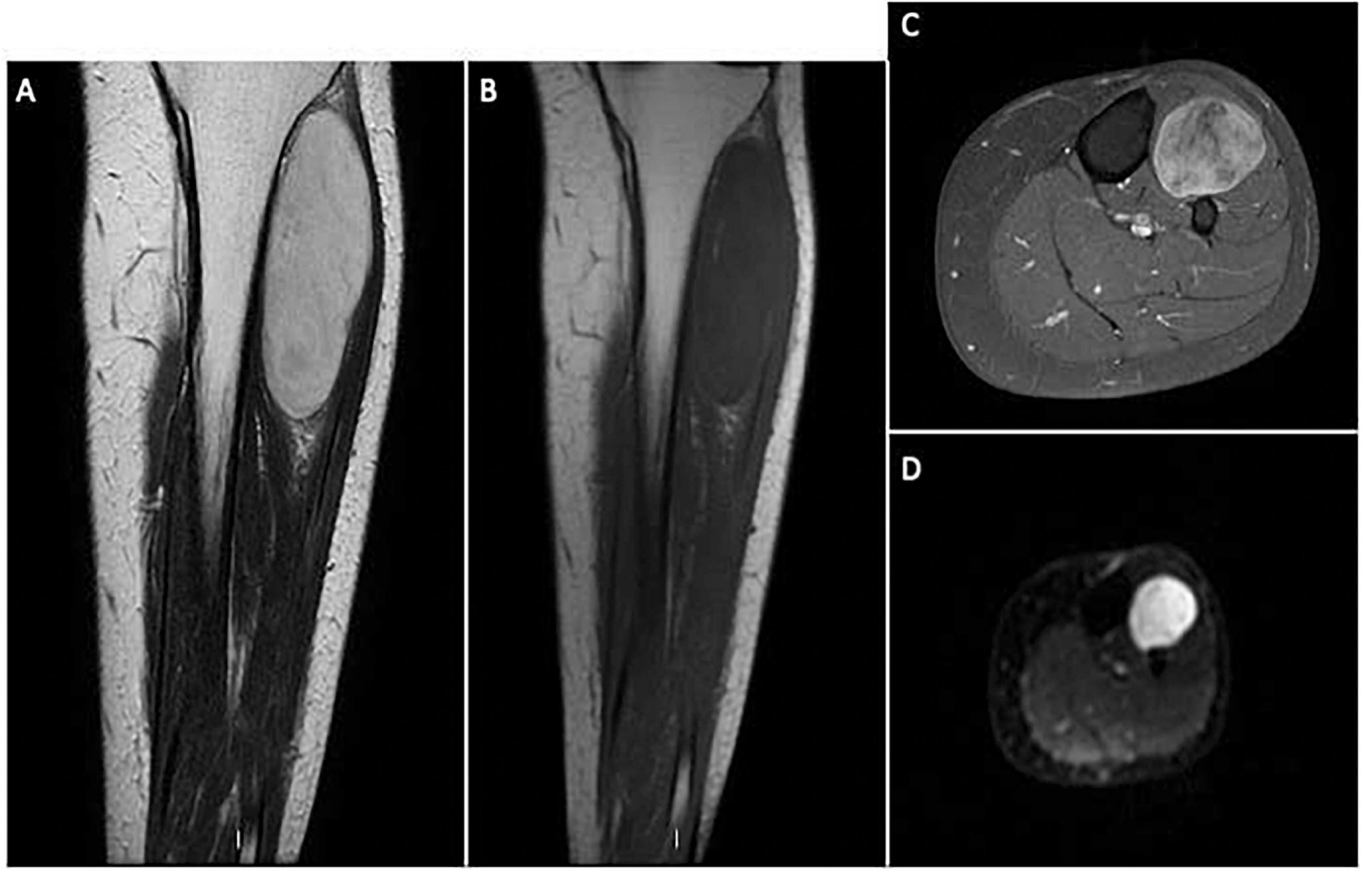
MRI of myxoid liposarcoma. (A) Coronal T2-weighted image; (B) Coronal T1-weighted image; (C) Axial T1 fat-saturated post-contrast image; (D) Diffusion-weighted image. The bulky solid mass shows oval morphology, a predominantly hyperintense signal on T2 with thin hypointense septa, a hypointense signal on T1, irregular diffusion restriction, and conspicuous post-contrast enhancement.
